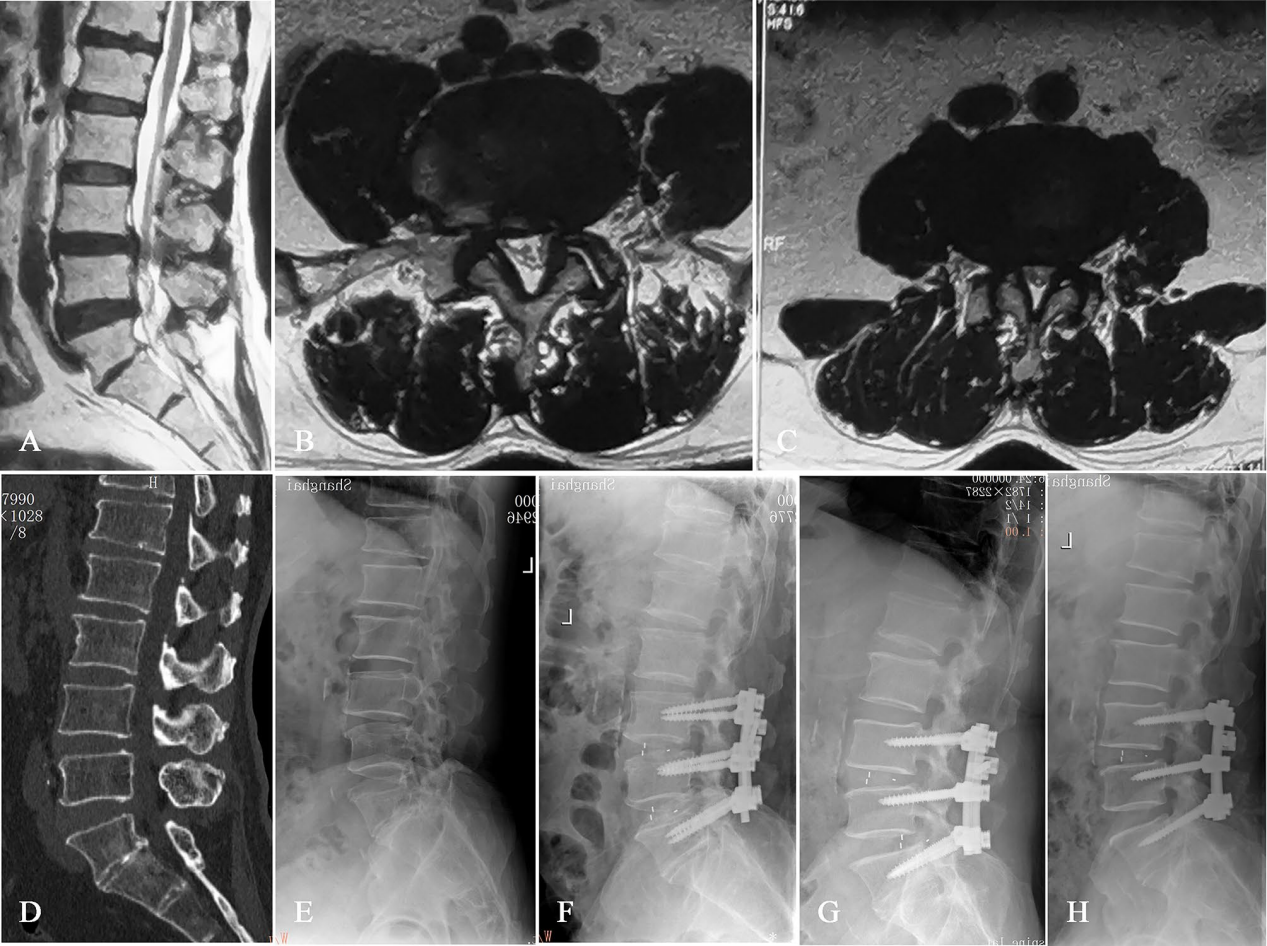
Imaging examinations (A-E) of a 66-year-old male showed lumbar spinal stenosis at L4/5 (B) and L3/4 (C) levels; Radiographic view of the patient at 3rd day (F), 3rd month (G) after first operation and after second operation (H)
